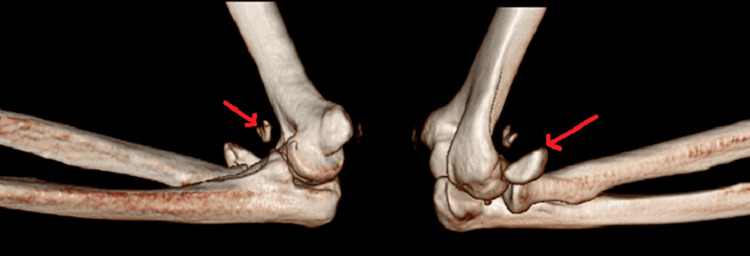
Three-dimensional reconstruction CT scan of the elbow joint showing avulsion fracture of the coronoid and fracture of the radial head (marked with red arrows) CT, computed tomography
